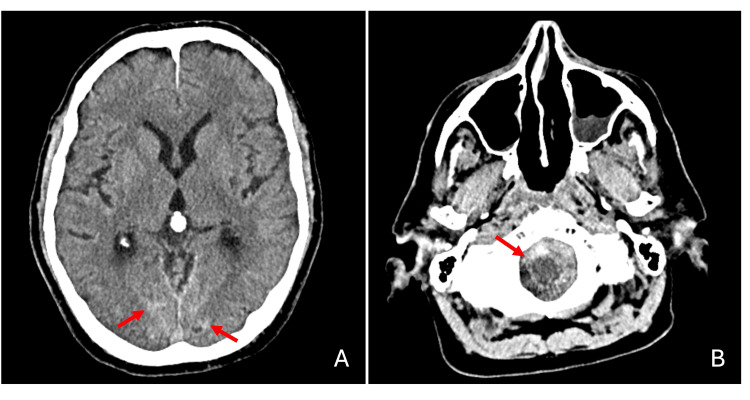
Brain CT. Mild subarachnoid hemorrhage within the posterior occipital sulci (A, red arrow), with a more significant distribution in the cisterna magna, predominantly along its anterior and left lateral aspects (B, red arrow), as previously documented on cervical MRI. CT, computed tomography; MRI, magnetic resonance imaging.
